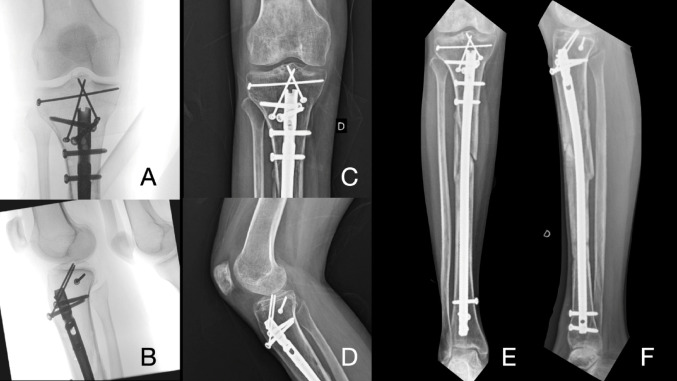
A, B Intraoperative fluoroscopic anteroposterior and lateral views of the knee demonstrating fixation of the tibial spine fracture with convergent retrograde screws, fixation of the sagittal tibial plateau component with a lag screw, and fixation of the diaphyseal component with a locked intramedullary nail. C, D Anteroposterior and lateral radiographs of the right knee obtained five months postoperatively, demonstrating union of the tibial spine fracture. E, F Anteroposterior and lateral radiographs of the right leg at five months showing bridging callus along the lateral cortex (AP view) and along the anterior and posterior cortices (lateral view), consistent with near-complete fracture healing
